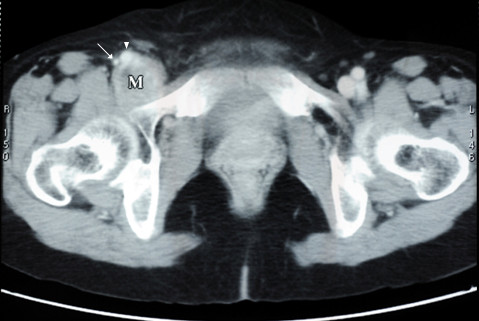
Pre-operative CT scan showing a pelvis mass (M) adjacent to the femoral artery (arrow) and femoral vein (arrowhead).
